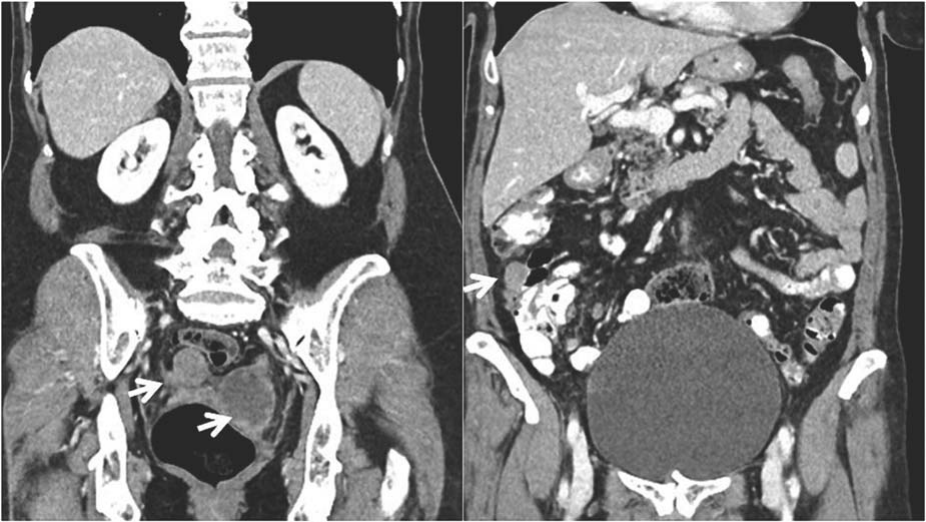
Coronal CT imaging showing the pelvic tumor implants and in the right colon.
